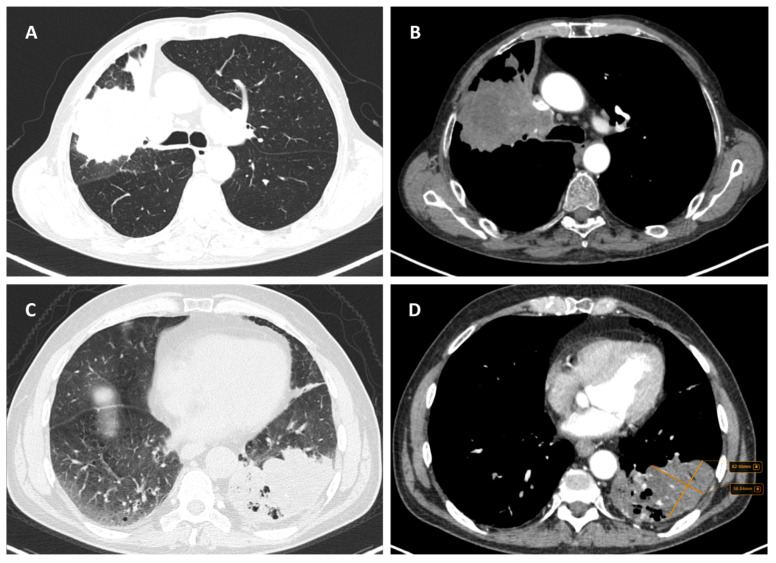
Axial CT of two different cases of adenocarcinoma: (A) a right peripheral mass (the maximal tumoral diameter over three centimetres) with spiculated margins, a small atelectatic area and hilar invasion is highlighted on lung window; (B) soft-tissue window that shows the same tumour as (A) with central necrosis and pleural invasion, including the main right bronchus; and (C,D) a peripheral mass with adjacent pneumonitis in the left lower lobe.
